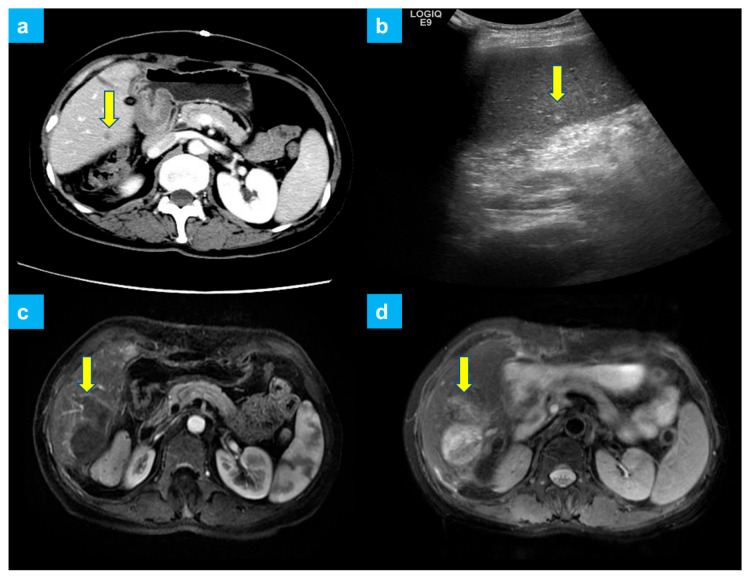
A case of TA for recurrent CRLM. (a,b) One month after resection of multiple intrahepatic metastases, ultrasound and enhanced CT showed a new low-density nodule in the right posterior lobe of the liver, with blurred edges, raising suspicion of metastases (yellow arrow). (c,d) Two months after TA, the enhanced MRI showed an abnormal mass signal shadow in the surgical area of the right lobe of the liver. No obvious enhancement was found on the enhanced scan (yellow arrow).
